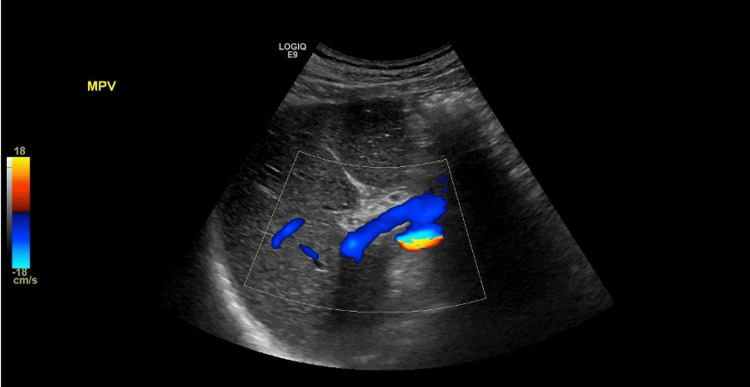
Ultrasound Doppler showing portal vein thrombosis
